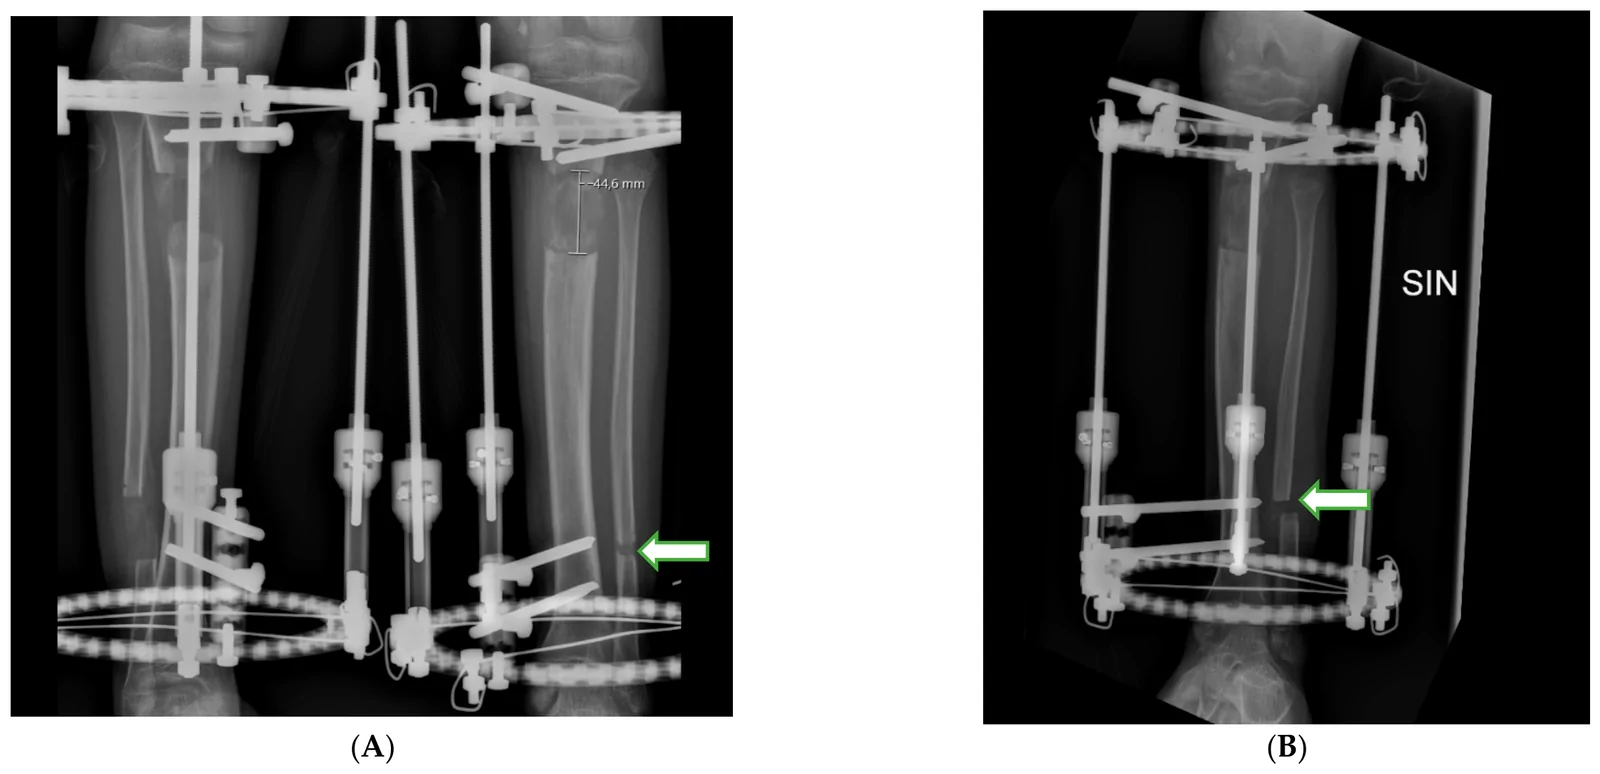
(A) X-ray showing premature consolidation of the left fibula compared to the contralateral, as indicated by the white arrow. (B) X-ray showing the outcome of the calloclasis on the left fibula of the patient, as indicated by the white arrow.
